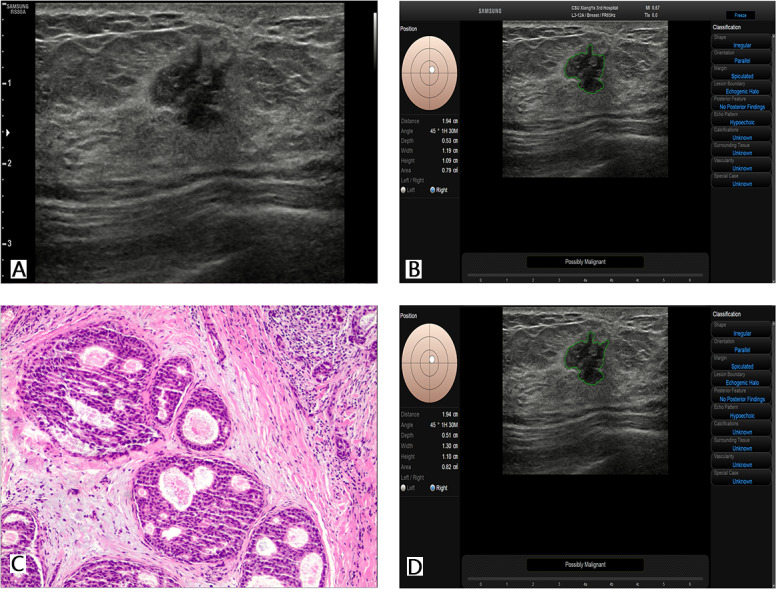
Case 2: a woman aged 43 years presented with a self-detected right breast mass. Ultrasound revealed a hypoechoic lesion measuring approximately 1.30 × 1.09 cm in the glandular layer of the upper inner quadrant of the right breast, adjacent to the areola. The lesion exhibited indistinct margins, an irregular shape, and a nonparallel orientation. No significant posterior acoustic attenuation was observed, whereas architectural distortion of the surrounding tissue was present (A). The initial S-Detect assessment suggested malignancy (B), and the repeated assessment yielded consistent findings (D). Postoperative pathology confirmed invasive ductal carcinoma with focal necrosis (C).
